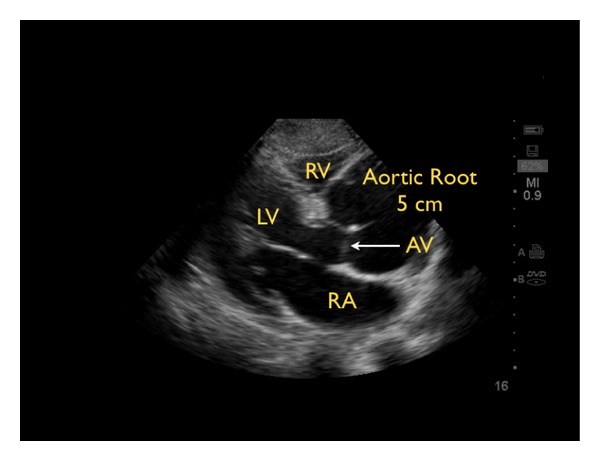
Aortic arch dissection with widened aortic root, parasternal long axis view. RV: right ventricle, LV: left ventricle, LA: left atrium, AV: aortic valve.
